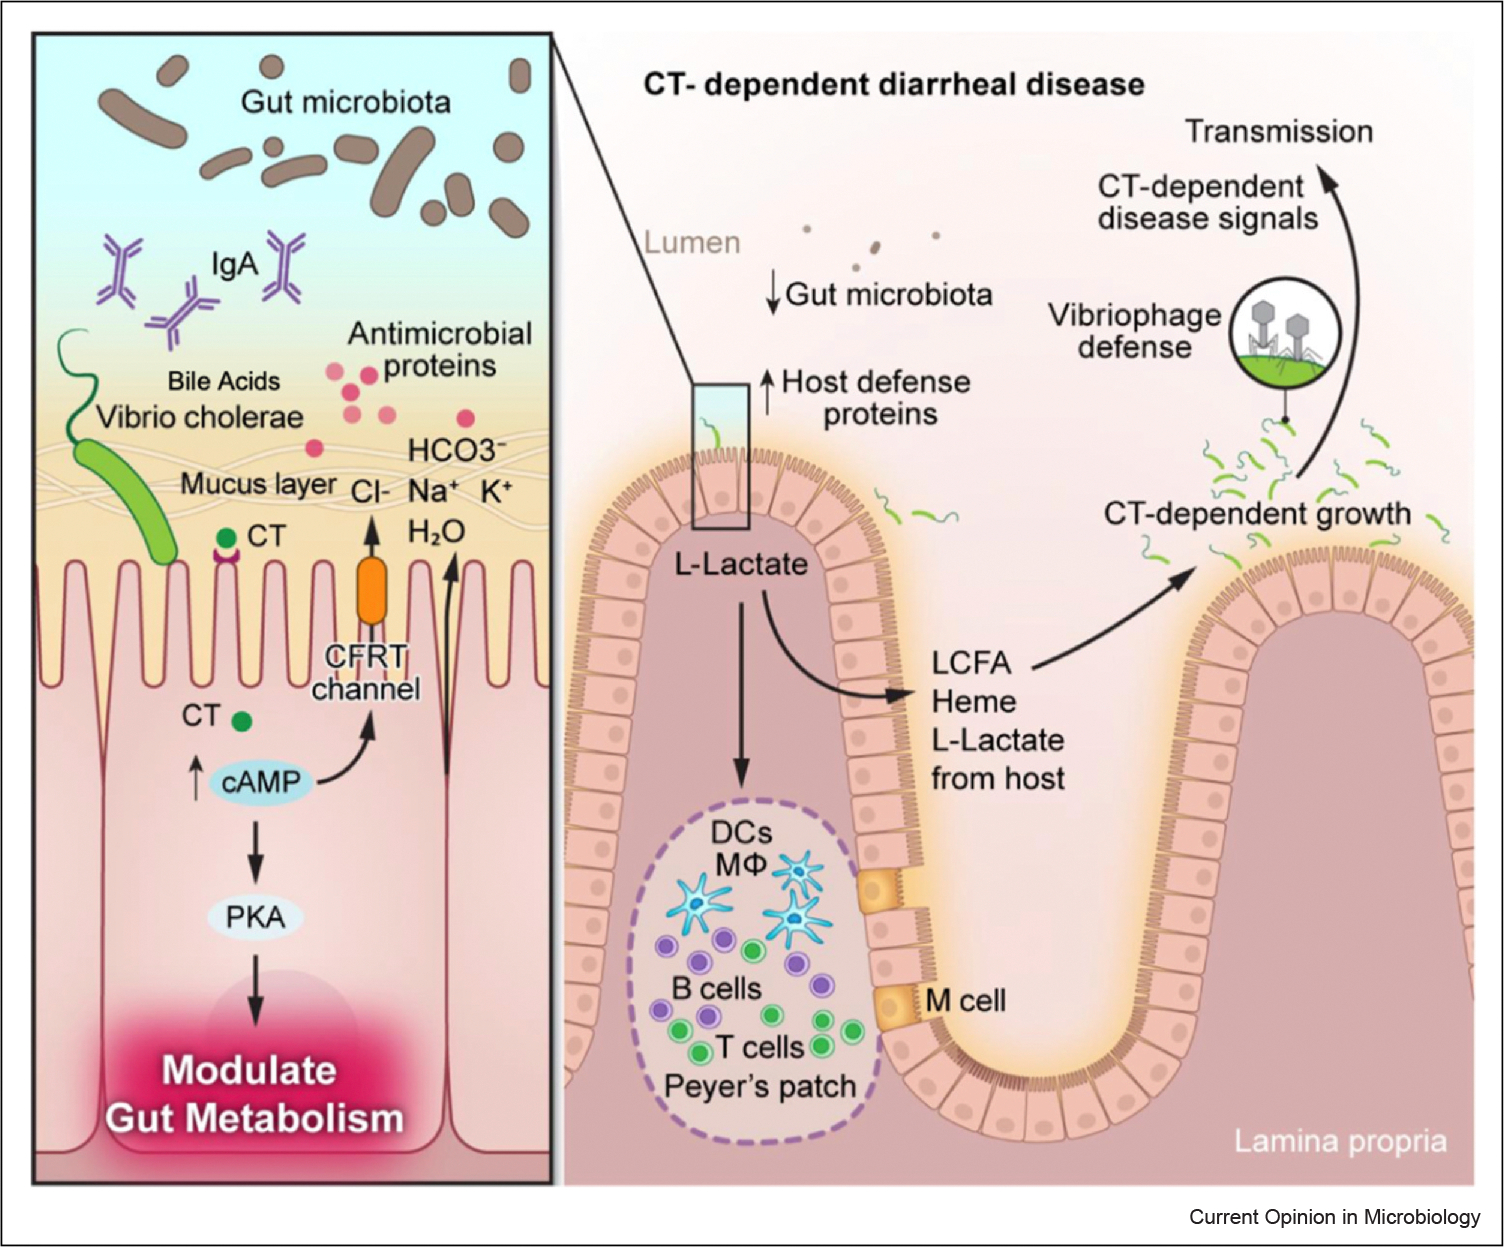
Host–pathogen interactions and metabolism during *Vibrio cholerae* infection. During infection, *Vibrio cholerae* colonizes the ileum of the SI and produces high levels of CT, which activates adenylate cyclase, increasing cellular levels of cAMP. Increased concentrations of cAMP lead to activation of PKA, which phosphorylates and activates the CFTR to secrete chloride ions out of epithelial cells, resulting in an electrolyte imbalance and massive water loss. Increased cAMP and activation of PKA leads to metabolic changes in target cells, which are predicted to lead to the secretion of host-derived LCFAs, L-lactate, and glycerol. During early stages of colonization (left side), *V*. *cholerae* encounters host factors, including bile acids, which act as signaling molecules to activate ToxT-dependent virulence factor expression. *V*. *cholerae* has to overcome components of the innate immune system, including antimicrobial proteins and secretory antibodies, such as IgA, after penetration of the mucus layer. The microbiota in the SI likely imposes ‘colonization resistance’ that *V*. *cholerae* overcomes by unknown mechanisms. The gut microbiota also may compete with *V*. *cholerae* for host-derived nutrients during CT-induced disease that the pathogen overcomes by evolutionary adaptation of the diseased gut as well as its T6SS. CT-induced disease leads to increased secretion of host defense proteins, which the pathogen overcomes by unknown mechanisms. CT-induced modulation of host metabolism promotes the luminal growth of *V*. *cholerae* during infection by acquisition of host-derived nutrients, including LCFAs, L-lactate, and heme. Host-derived L-lactate that is produced during CT-induced disease may play a role in ‘lactylation’ of immune cells in the gut. Furthermore, we propose that CT-induced growth of *V*. *cholerae* plays a role in pathogen susceptibility to phage during infection that the pathogen overcomes by deploying phage defense systems. Altogether, these complex chain events that lead to CT-mediated disease and successful replication of *V*. *cholerae* have been selected for during the evolution of the pathogen in order to ensure fecal–oral transmission of a new host.
